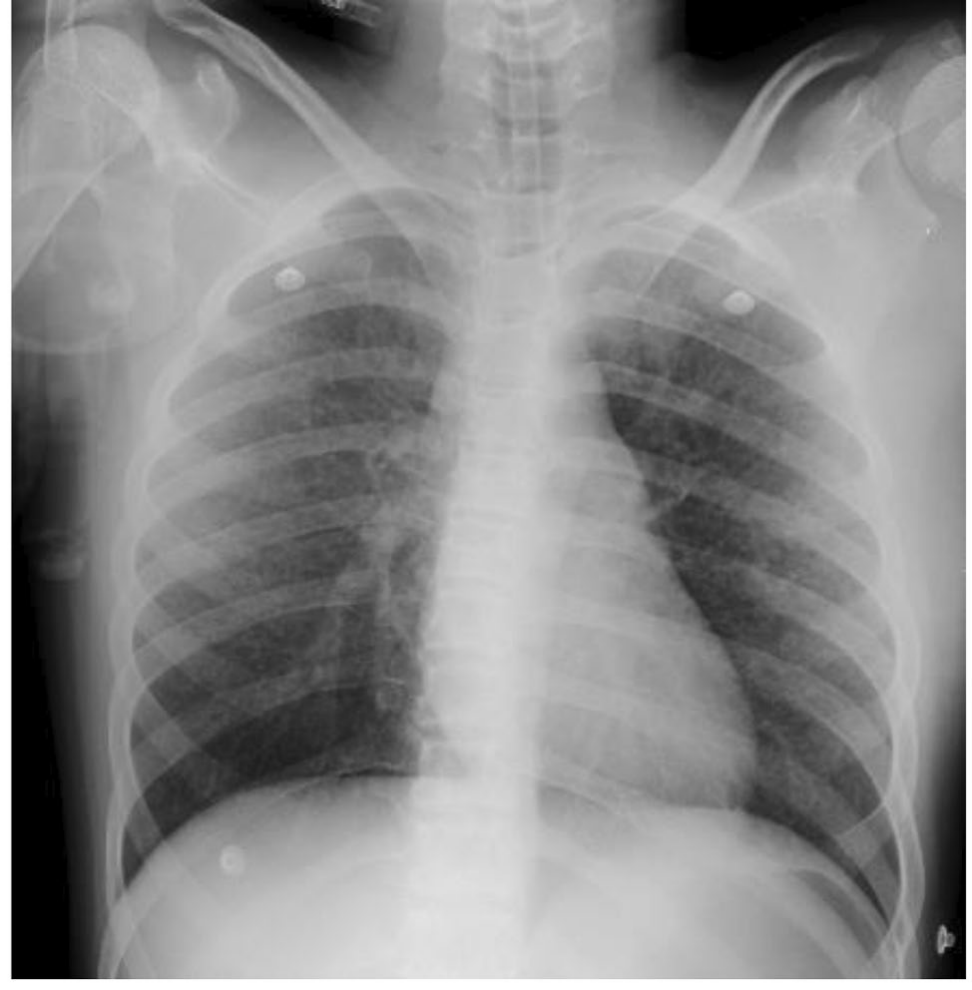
CXR. PICU admission: AP chest x-ray demonstrates wide spread tiny nodular opacities distributed throughout both lungs
